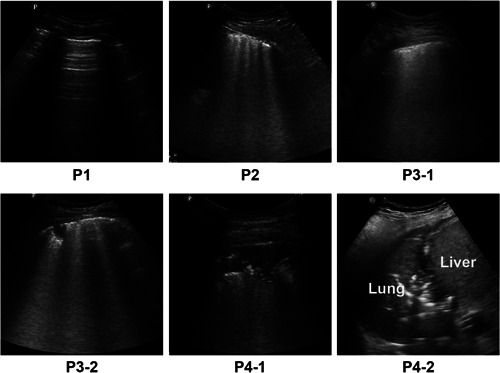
Four types of ultrasound scoring features, P1 normal ventilation (N‐type): the ultrasonographic features showed A‐line sign or ≤2 independent B lines, indicating good lung inflation. P2 (B1‐type): Multiple B lines, with an interval of about 7 mm between the B lines (B7 lines). P3‐1 and P3‐2 (B2‐type): Multiple B lines, with an interval ≤3 mm between the B lines (B3 lines), with or without small patchy consolidation. P4‐1 and P4‐2 (C‐type): The ultrasonographic features were hepatization or fragment sign of lung tissue, with dynamic bronchial inflation sign, with or without a small amount of pleural effusion, indicating consolidation of the lung. N = 0, B1 = 1, B2 = 2, C = 3.
